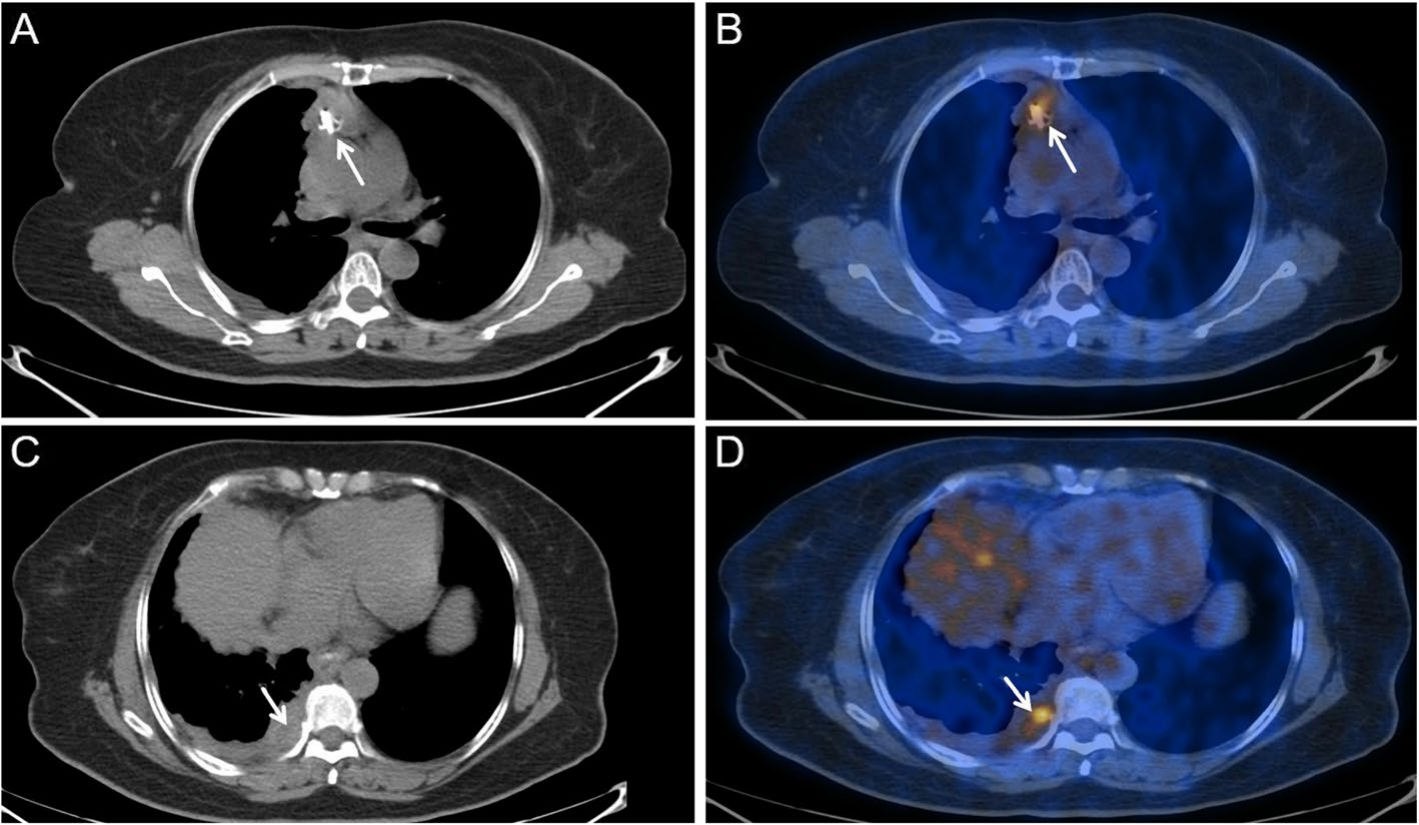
PET/CT findings of a 62-year-old patient with type B3 thymoma. A Right anterior medial malleolus soft tissue mass shadow, adjacent blood vessels were not clear and calcified calcification inside the tumor was observed (arrow). B A high metabolism signal (arrow) was seen in the tumor, SUVmax $$=$$ 3.9. C A small amount of fluid in the right thoracic cavity (arrow). D Right pleural hypermetabolism nodules (arrow), SUVmax $$=$$ 2.5
